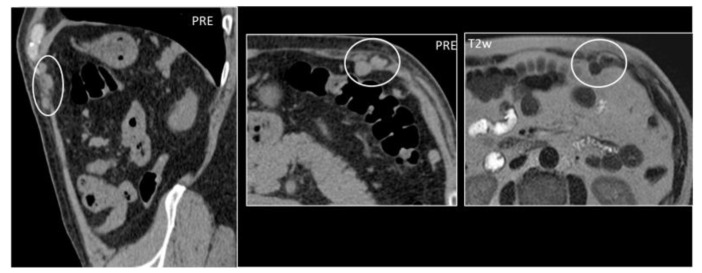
A 59-year-old woman who underwent splenectomy some years prior. During a CT scan, new, rounded masses (circles) were found along the peritoneum and the left rectus abdominis muscle. These formations show parenchymal attenuation on CT (pictures 1 and 2) and share the same T2 intensity as the spleen. These lesions were later characterized as splenosis.
